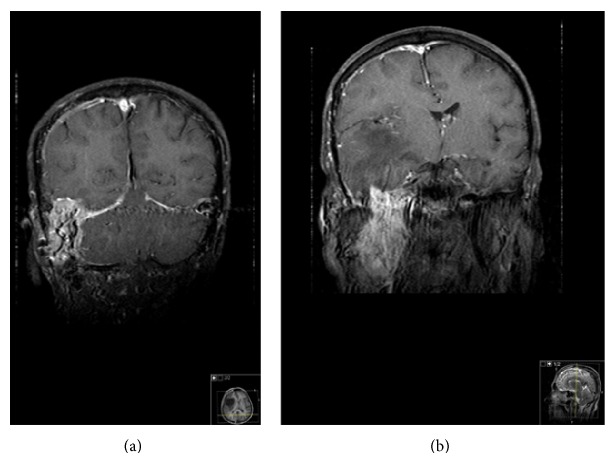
Cranial magnetic resonance images demonstrate (a) a mass lesion that arises from the right jugular foramen, extending to the medial cranial fossa, consistent with glomus jugulare, and (b) a mass lesion at the carotid bifurcation, consistent with glomus caroticum.
